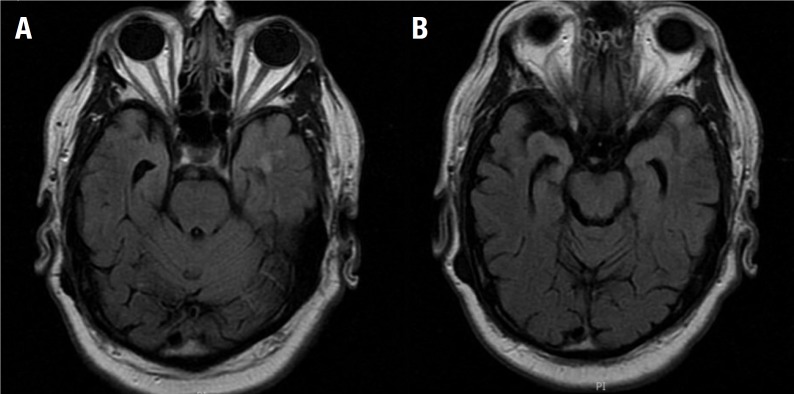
Brain Magnetic Resonance Imaging FLAIR sequence revealing elevated signal intensity in left mesial temporal region [A] and in left temporal pole [B].
